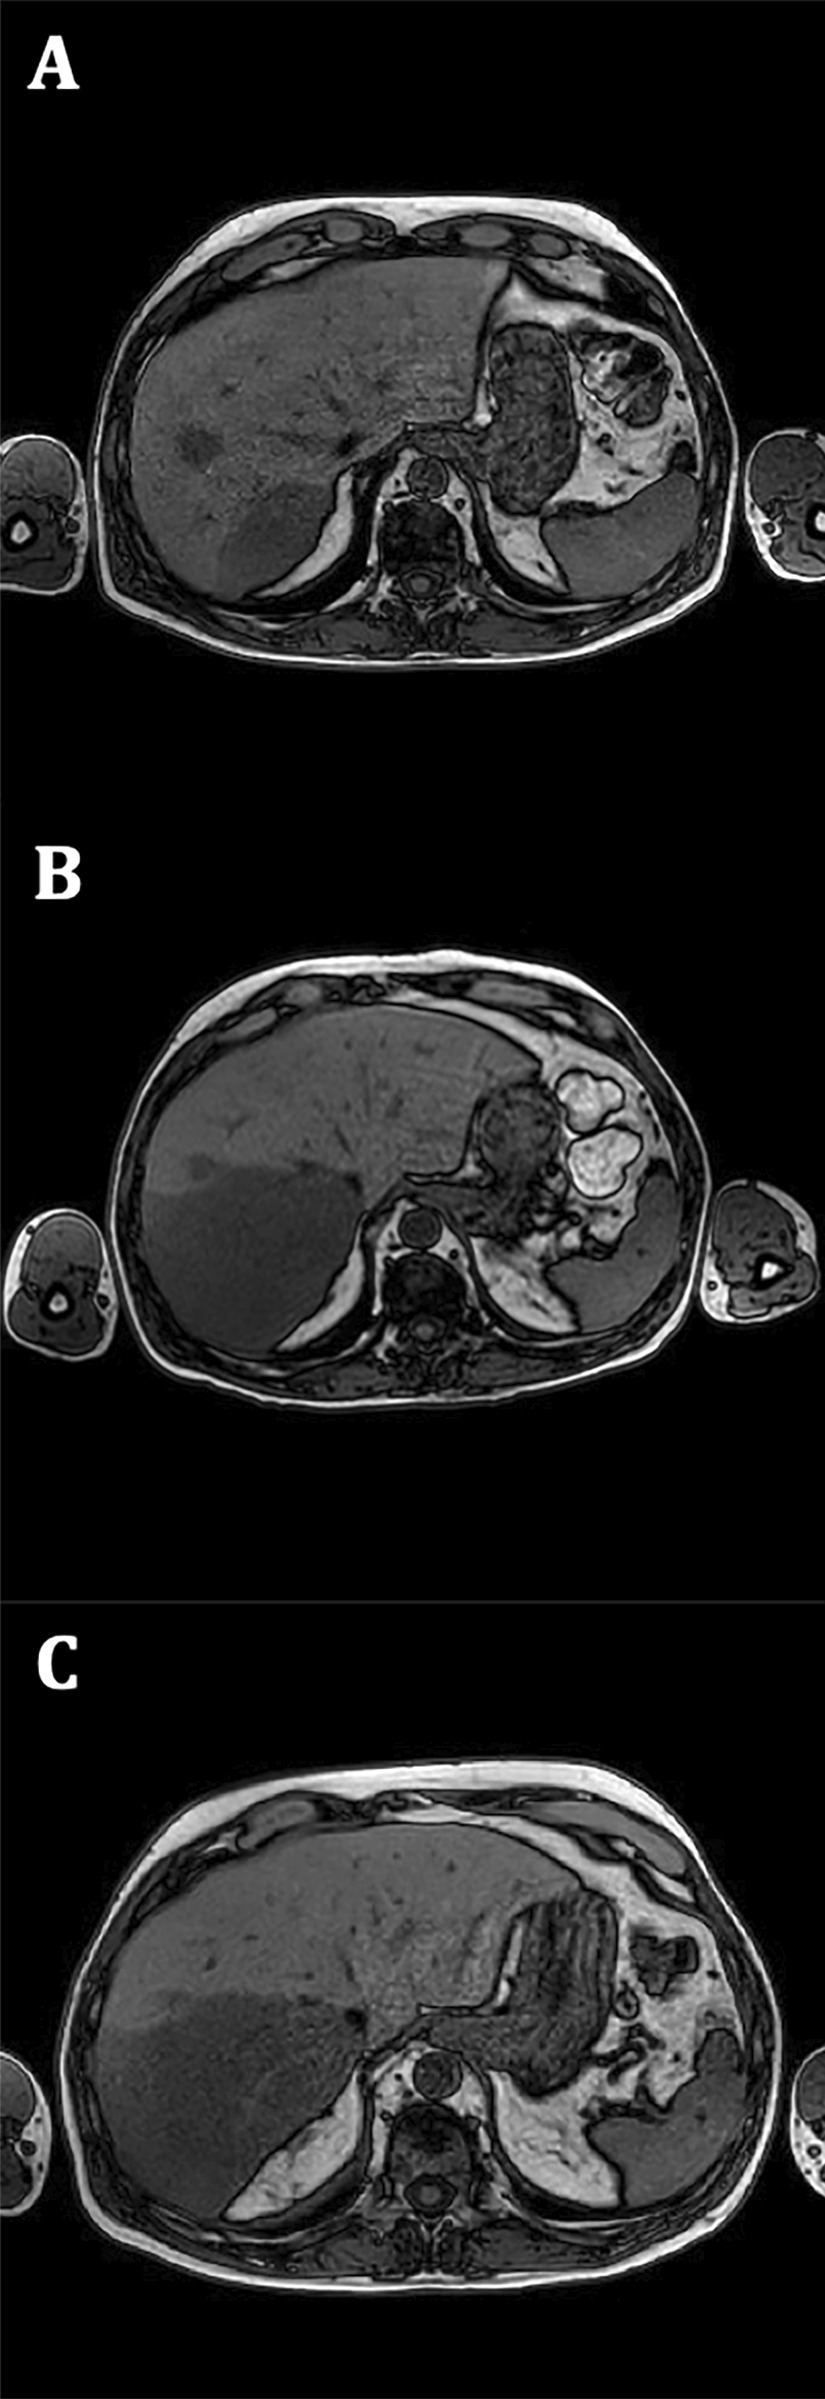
A Initial MRI showing liver spread of the disease (March 2018). Hepatic lesions were identified in segments VII and VIII of the liver. B Progression of the hepatic lesions. C Stability of the hepatic lesions under venetoclax treatment (August 2018)
